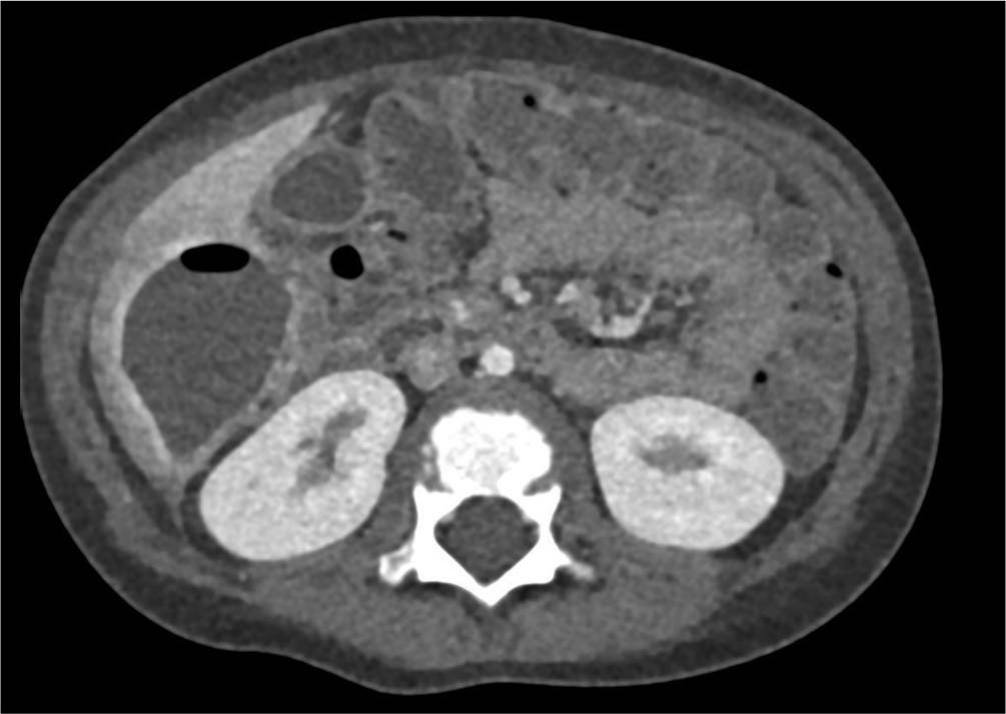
CT axial view of a subhepatic abscess. Contrast-enhanced capsule and fluid-air level visible.
